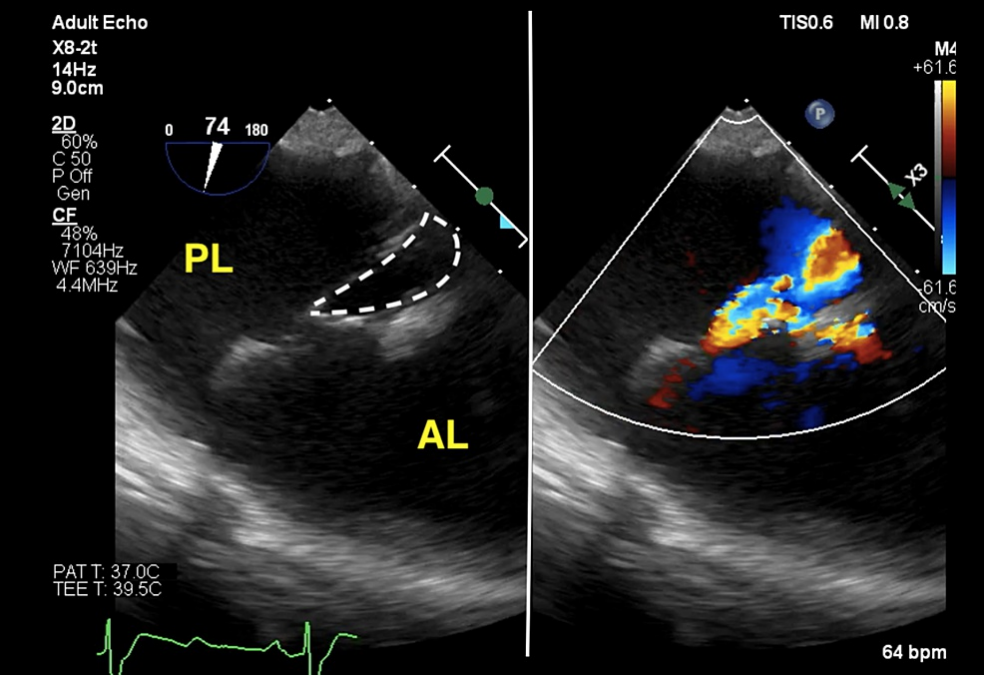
Transgastric TV short axis view from intraoperative TEE. Severe tricuspid regurgitation from the septal leaflet side, characteristic of Ebstein’s anomaly, can be observed. The dotted line indicates the area corresponding to septal leaflet defect. AL: anterior leaflet; PL: posterior leaflet; TEE: transesophageal echocardiography; TV: tricuspid valve
